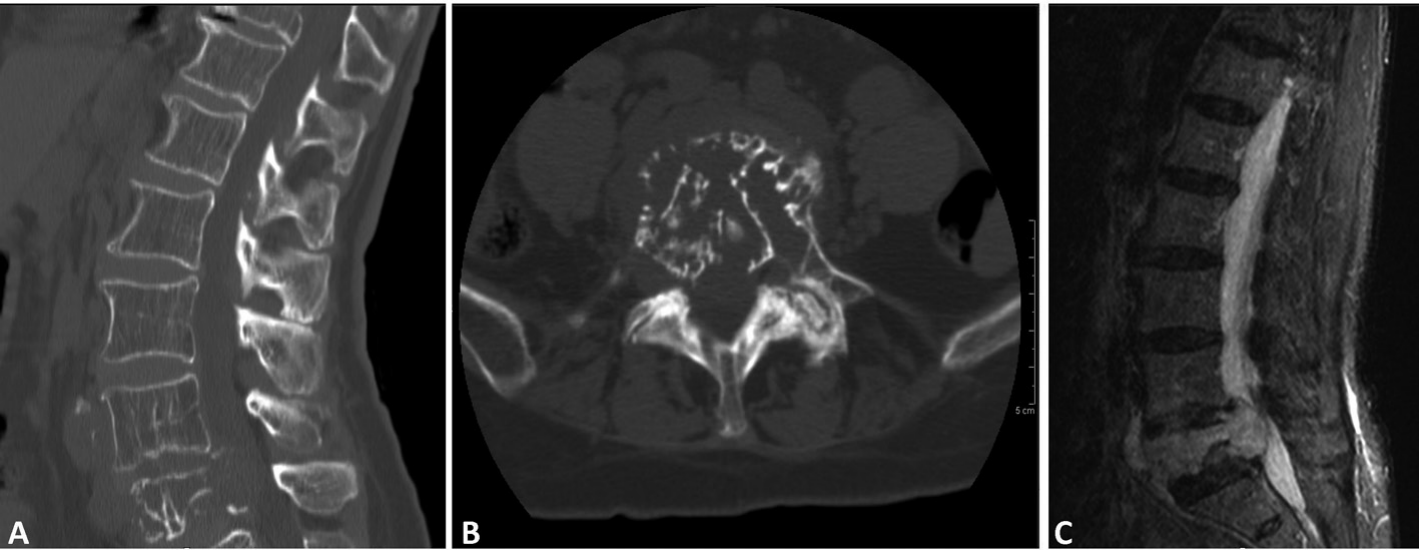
(A); (B); (C) (CT - sagittal, axial and MR T2- sagittal) soft tissue lesion extending both anteriorly and posteriorly causing canal stenosis, and destructed vertebral body, with canal involvement.
